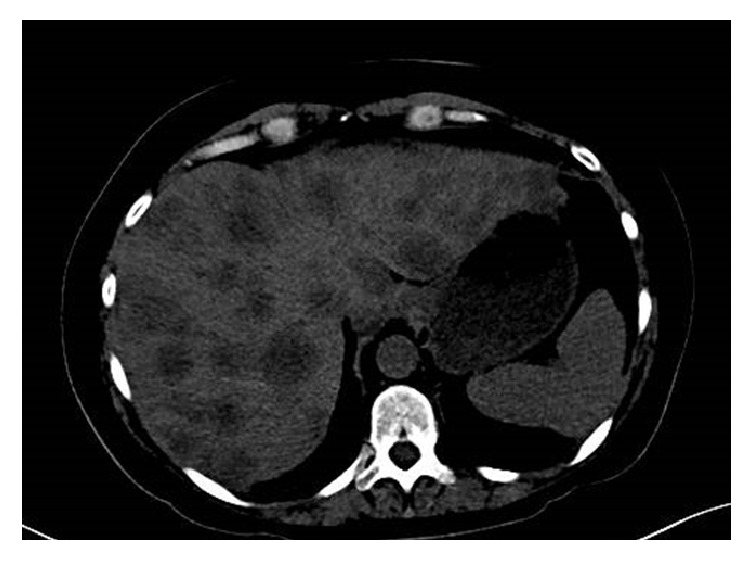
CT scan of the abdomen at the time of recurrent disease demonstrated innumerable lesions in the liver.
